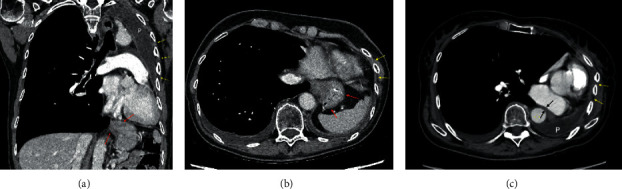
Coronal (a) and axial (b, c) reformats on contrast-enhanced chest CT more saliently depict the leftward cardiomediastinal shift and ipsilateral intercostal space narrowing (yellow arrows). Expected postsurgical fluid filling the pneumectomy space is present only along the superoposterior left hemithorax (labeled P). A small hiatal hernia is present (red arrows), which may be partly due to the tortuous course of the esophagus in the left hemithorax and consequent upward traction on the stomach. There may be some extrinsic compression on the esophagus (black arrows) between the left atrium and descending thoracic aorta, although an obstruction or poor distensibility was suggested on esophagram.
